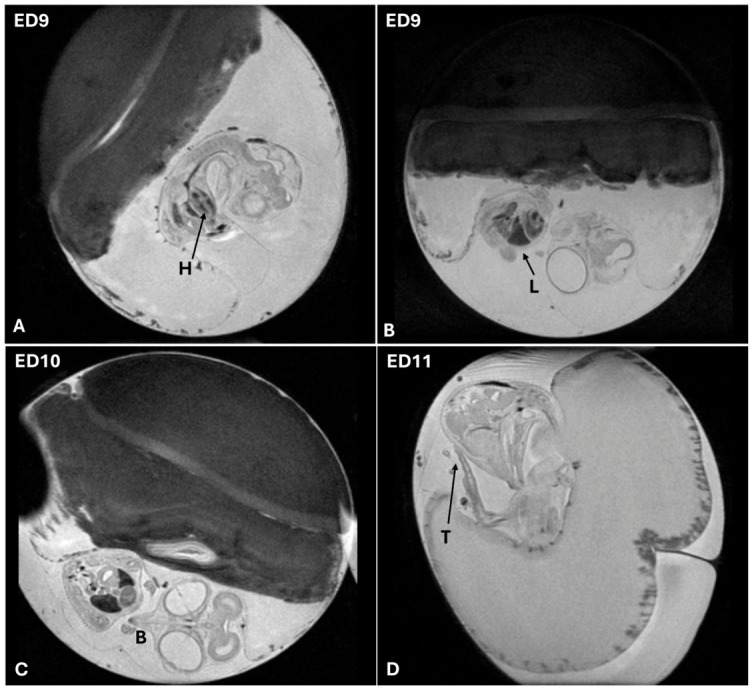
(A–D) T2-weighted MRI images of CE between ED9 and ED11 showing reduction in yolk sac and albumen volumes. Images acquired in sagittal and paracoronal planes. Increased anatomical detail is evident, including a more defined beak, visible liver within the thoracic cavity, femur growth, and measurable third toe. Abbreviations: H, heart; L, liver; B, beak; T, third toe.
